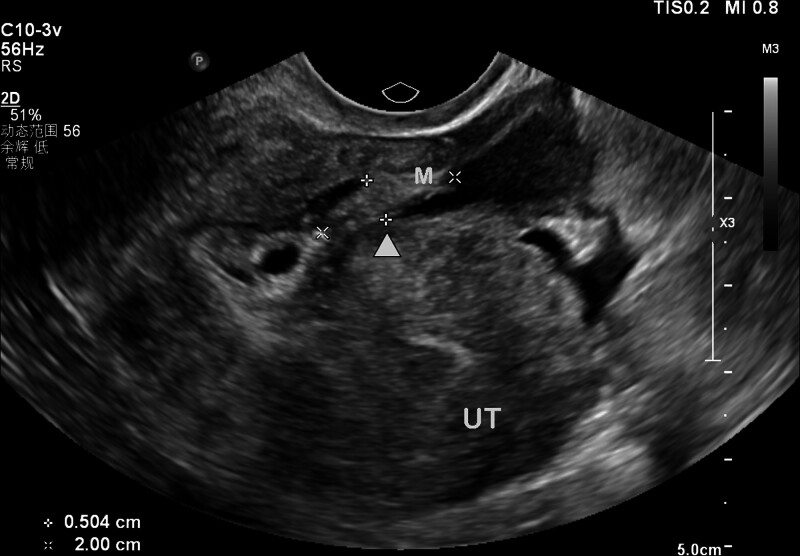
Transvaginal ultrasound demonstrates a 2.00 × 0.50 cm hypoechoic mass (*) originating from the right parauterine region, with intramyometrial extension. Arrow indicates the interface between parauterine and myometrial components. UT = uterus, M = mass.
